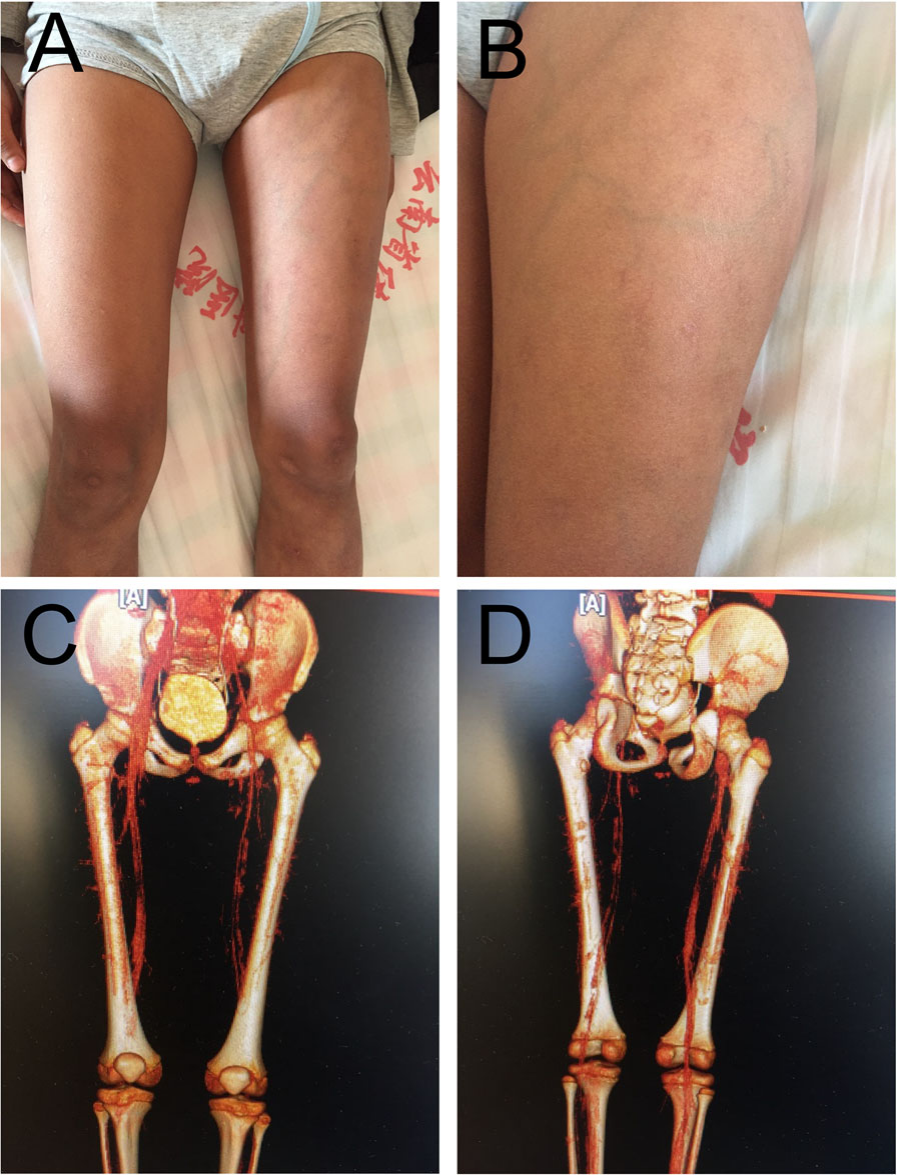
Clinical and Vascular CT three-dimensional reconstruction images of the patient. a, b. His left lower extremity thigh was inflamed and swollen with varicose veins. c, d. Vascular CT three-dimensional reconstruction showed left lower extremity showed extensive mural thrombosis
